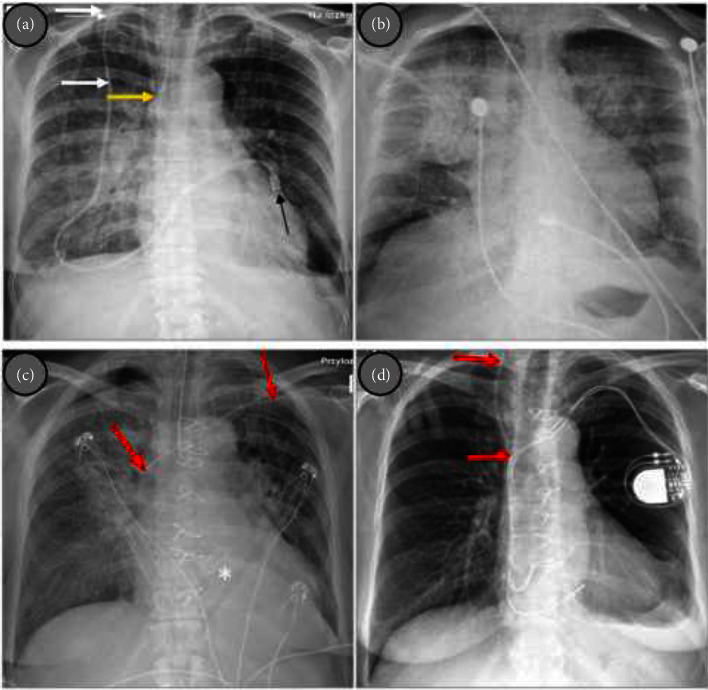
Chest x-ray: pulmonary congestion and bilateral pleural effusion; white arrows: tunneled part of the Broviac catheter, yellow arrow: catheter in the superior vena cava—SVC, black arrow: catheter's cap (a), few hours later, after Broviac catheter removal; see progression of the pulmonary congestion to pulmonary edema (b), the day after aortic valve replacement with bioprosthesis—AVB—white asterix (c), and after pacemaker implantation (d). Red arrows—new (percutaneous) central venous catheter inserted into the SVC directly through the skin via left subclavian vein and then via right internal jugular vein.
